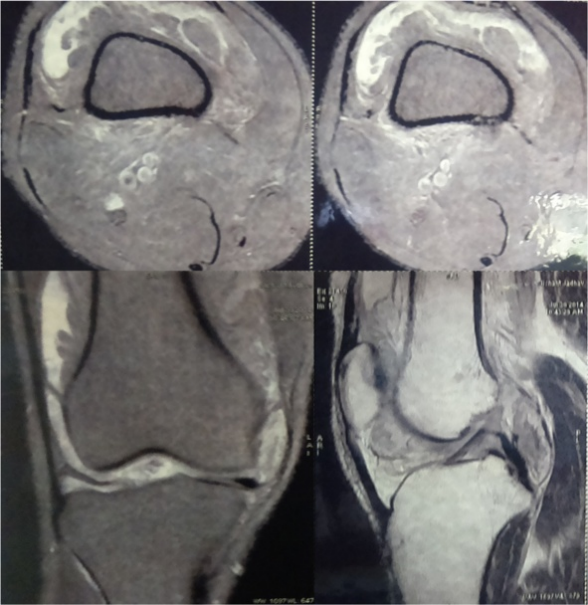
MRI Image of Knee showing Frond-like synovial proliferative growth (Case 1) clockwise from top left (transverse image showing synovial proliferation around distal femur; transverse image showing synovial effusion in suprapatellar pouch; coronal image showing frond like proliferation both medially and laterally; sagittal image showing involvement of patellofemoral space and posterior capsular region
